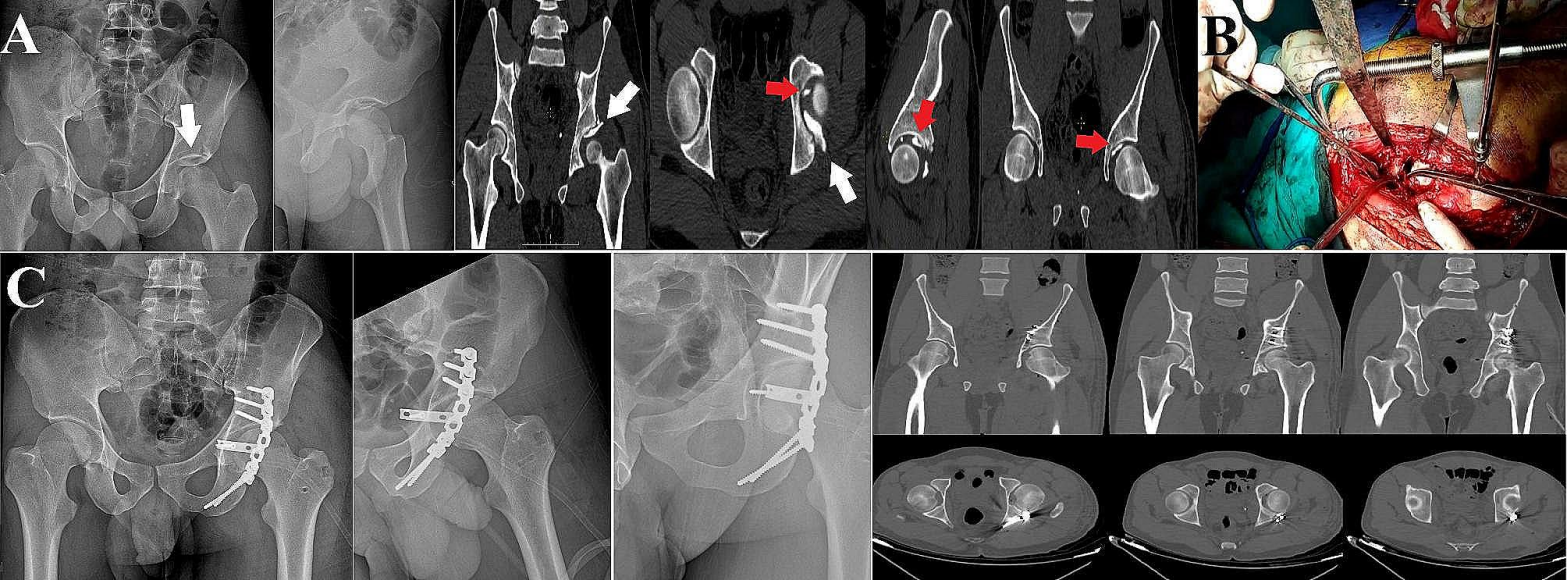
A male patient, 38 years old, presented with a posterior wall fracture of the left acetabulum. A, preoperative plain radiographs and CT scans showing the intraarticular incarcerated posterior wall fragment (white arrow) and a loose intraarticular fragment (red arrow). B, an intraoperative image showing the femoral distractor assisted hip joint distraction, which helped retrieve the posterior wall incarcerated fragment. C, post-operative plain radiographs and CT scans showing fracture anatomical reduction and clearance of the hip joint space
